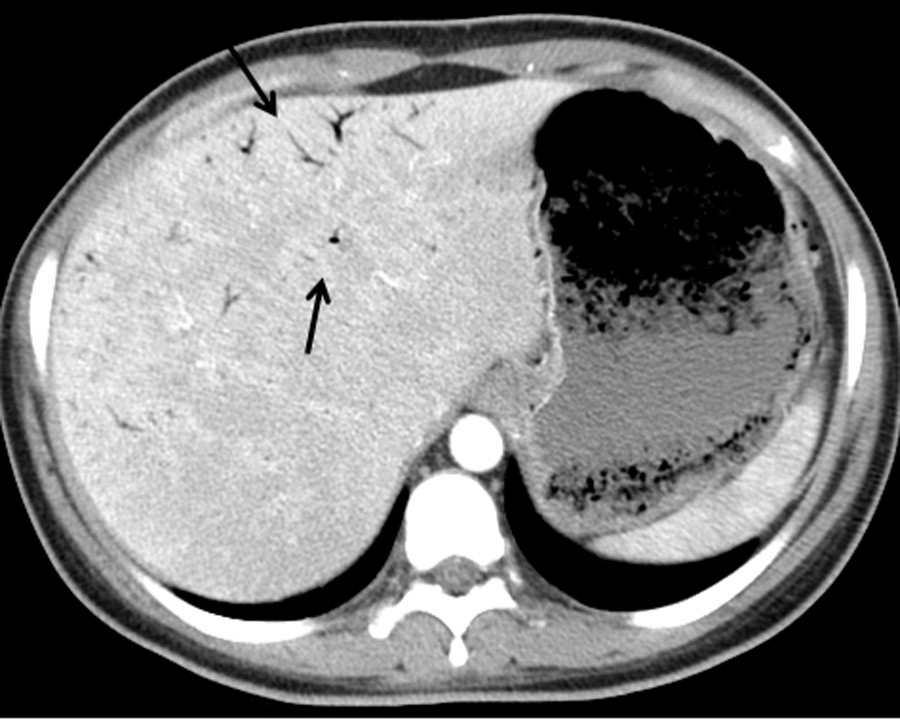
Abdominal computed tomogram with arrows pointing to air in the portal tract and bubbles outlining the stomach.
